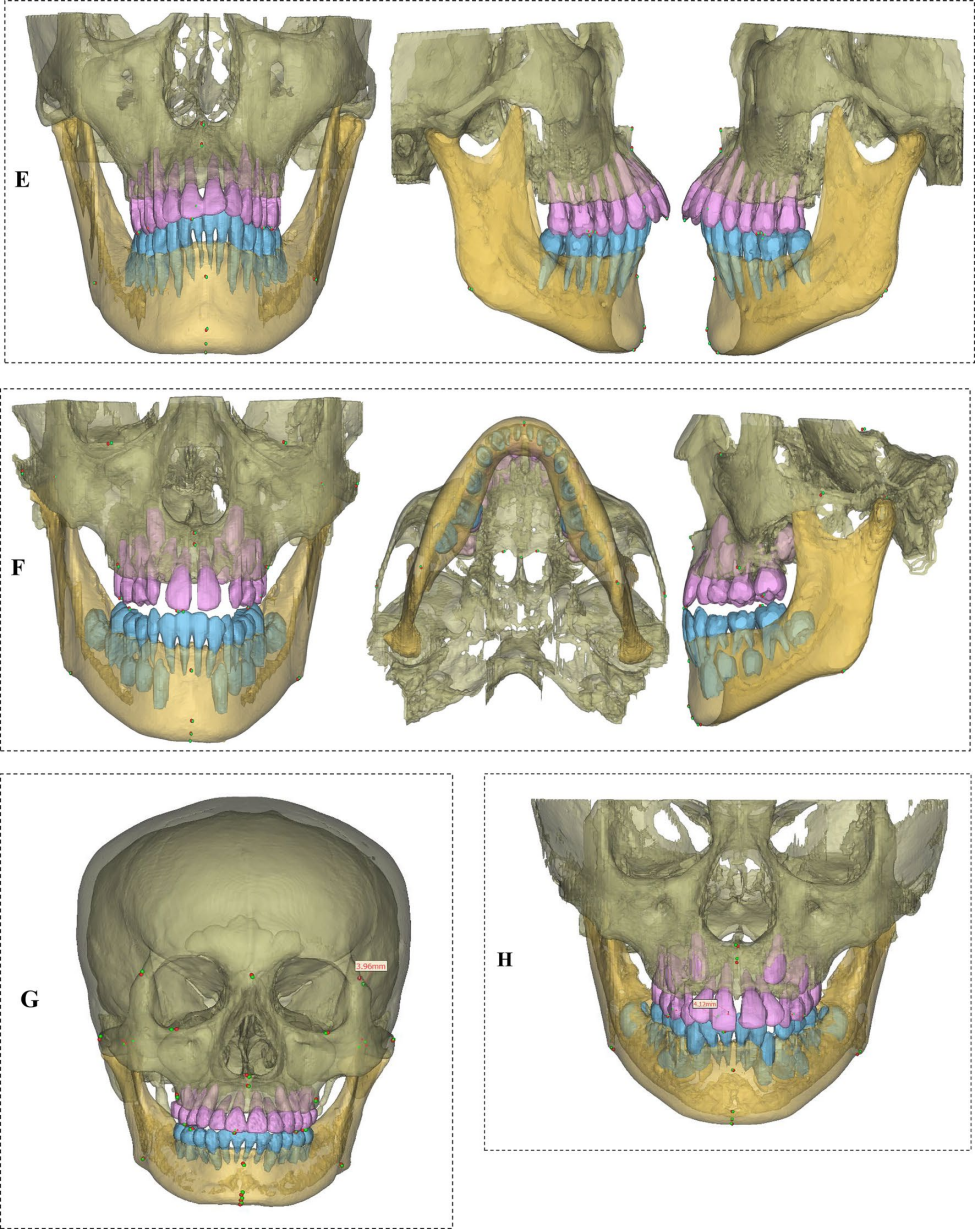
Frontal, bottom, and left views of the three-dimensional models of SCT and CBCT. Red landmarks represent the reference standard, and green ones are the predicted landmarks. *M* malocclusion, *MDL* missing dental landmarks, *MA* metal artifacts. A (MDL): SCT sample with the first molar on the left side of the mandible missing, where the L6L landmark was absent, with an MRE of 1.41 mm for all displayed landmarks. B (M + MA): In this SCT sample exhibiting crossbite, a porcelain crown was present on the left upper premolar. The landmarks primarily impacted by these artifacts were the UI and LI, with localization errors of 1.24 mm and 1.26 mm, respectively. C (M): SCT malocclusion sample, with an MRE of 1.34 mm for all displayed landmarks. D (MDL): CBCT sample with tooth absence, featuring a landmark MRE of 0.98 mm. E (M): Skeletal malocclusion CBCT, with a landmark MRE of 1.01 mm. F (MDL): A CBCT sample from a 7-year-old child with tooth absence, featuring a landmark MRE of 0.94 mm. G (M): One case of malocclusion, with an average MRE of 1.47 mm on SCT. The largest error was at the FzL point (The most medial and anterior point of each frontozygomatic suture at the left orbital rim), with an error of 3.96 mm, which is the landmark with the largest error among all SCTs. H (M + MDL): One case of malocclusion with tooth loss in an 8-year-old child, with a landmark MRE of 1.18 mm on CBCT. The largest error was at the LI point (The most midpoint of the crown tip of the right lower central incisor), with an error of 4.12 mm, which is the landmark with the largest error among all CBCTs
